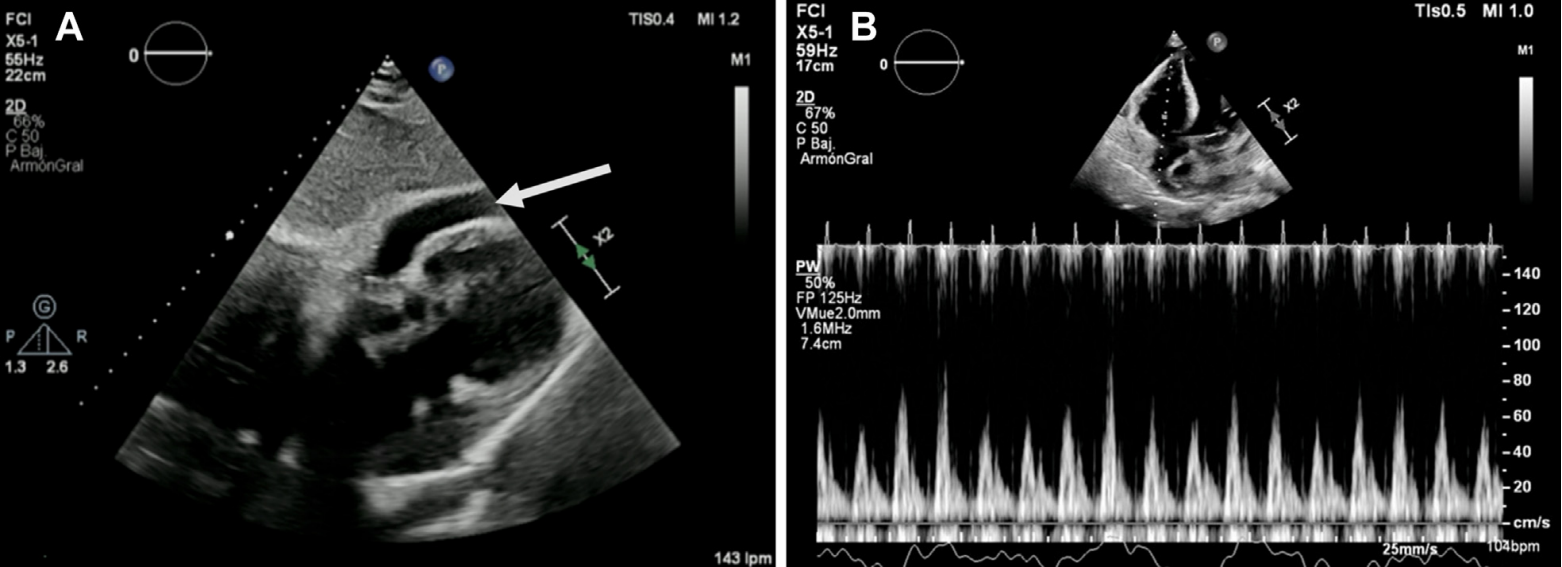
Transthoracic Echocardiogram (A) Moderate pericardial effusion (arrow) and (B) transtricuspid doppler evaluation showing marked inflow variability suggestive of increased intrapericardial pressures.
